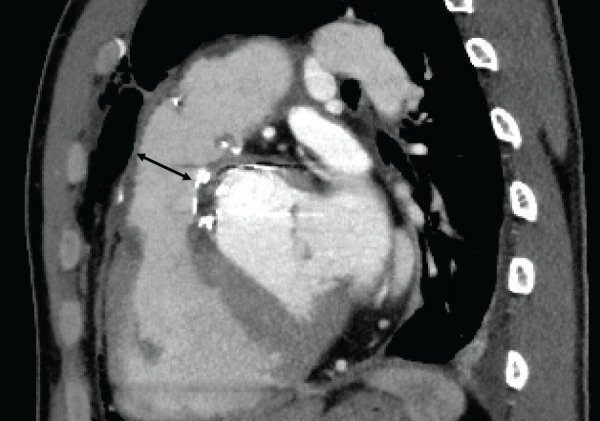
Postoperative CT image showing relief of RVOT stenosis, with the RVOT diameter increased to 22 mm (double-headed arrow). RVOT, right ventricular outflow tract
